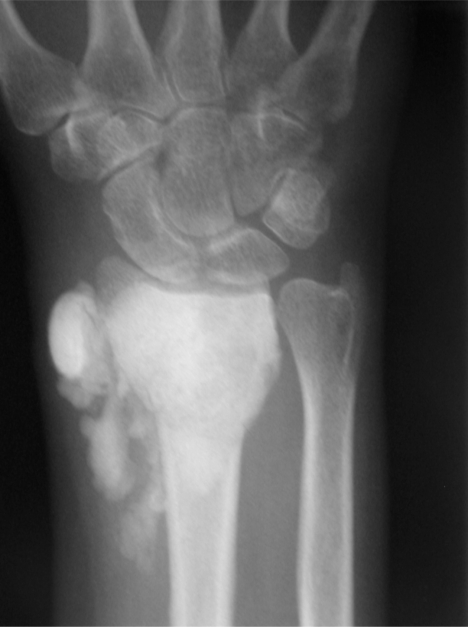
Post-operative AP radiograph of the wrist of a 51 year old woman with a giant cell tumor of the distal radius who underwent extended curetting and allograft packing. There is extensive bone graft material in the soft tissue.
